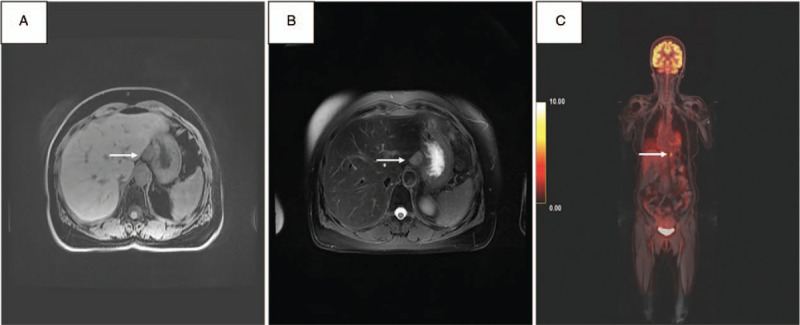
A–C, An axial PET-CT/MRI hybrid scan of the abdomen showed a well-circumscribed, homogenous mass in the gastrohepatic ligament. It was slightly (A) hyperintense on T1 imaging, (B) hyperintense on T2 imaging. C, An increased 18F-fluorodeoxyglucose uptake (SUVmax 7.4) can also be noted in the gastrohepatic ligament on PET-CT scan.
